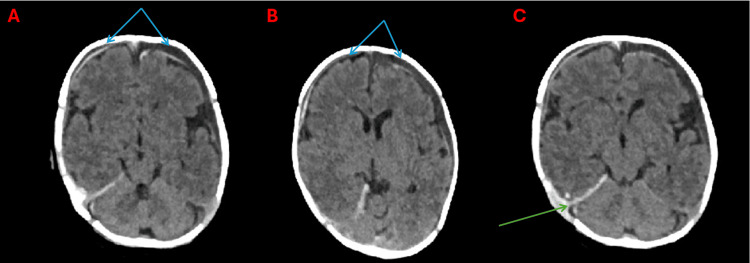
Non-contrast head CT, axial sections at three levels, brain window. Bilateral subdural hematohygromas in the frontal region (A and B, blue arrows). Bleeding along the right side of the cerebellar tentorium (C, green arrow).
